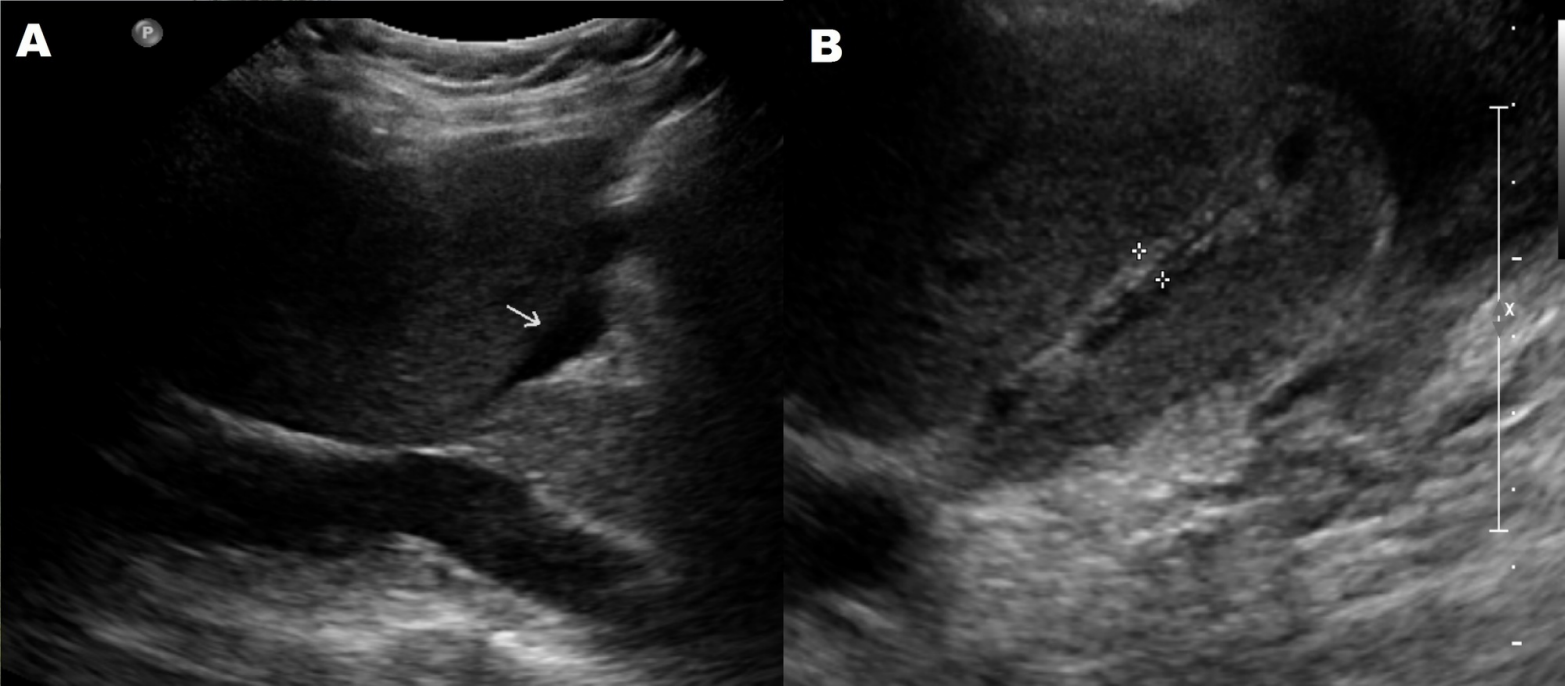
An abdominal ultrasound showing a distended gall bladder (GB) with pericholecystic fluid (A) and a thickened GB wall (B)
